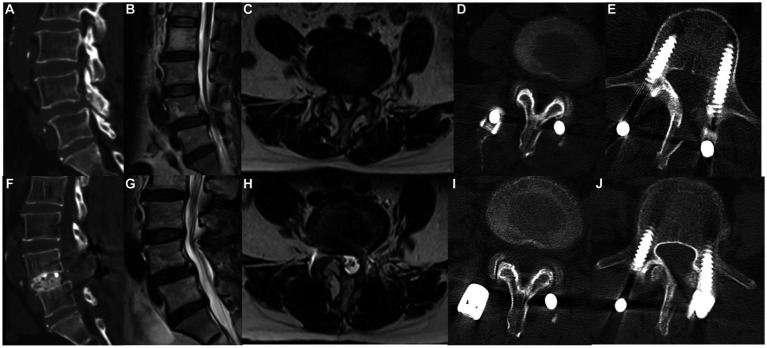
A 69-year-old man underwent MHSPS TLIF for L4/5 lumbar spinal stenosis with instability and unilateral lower extremity pain. Preoperative sagittal computed tomography demonstrates segmental instability (A). Preoperative sagittal (B) and axial (C) T2-weighted magnetic resonance imaging shows L4/5 and L5/S1 spinal stenosis. Axial computed tomography 5 days after surgery shows the split spinous process at two levels (D,E). Postoperative sagittal computed tomography demonstrates good interbody fusion (F). Sagittal (G) and axial (H) T2-weighted imaging 1 year after surgery shows sufficient decompression and no marked difference in the cross-sectional area of the paraspinal muscles. Axial computed tomography 1 year after surgery demonstrates a bony union of the spinous process at two different levels (I,J).
